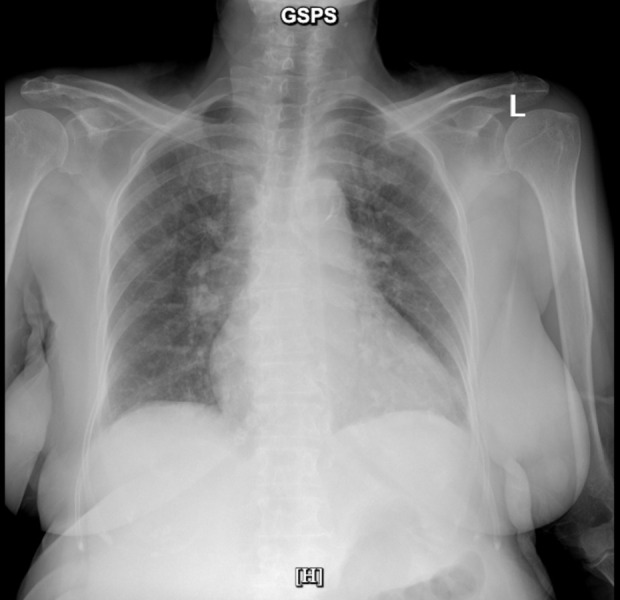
Clinical improvement following adjunctive acetazolamide therapy. Portable AP chest radiograph (08/12/2025): Marked interval clearing of alveolar opacities with resolution of pulmonary edema; cardiomegaly persists. Image obtained on hospital day 4, following three days of adjunctive acetazolamide therapy
